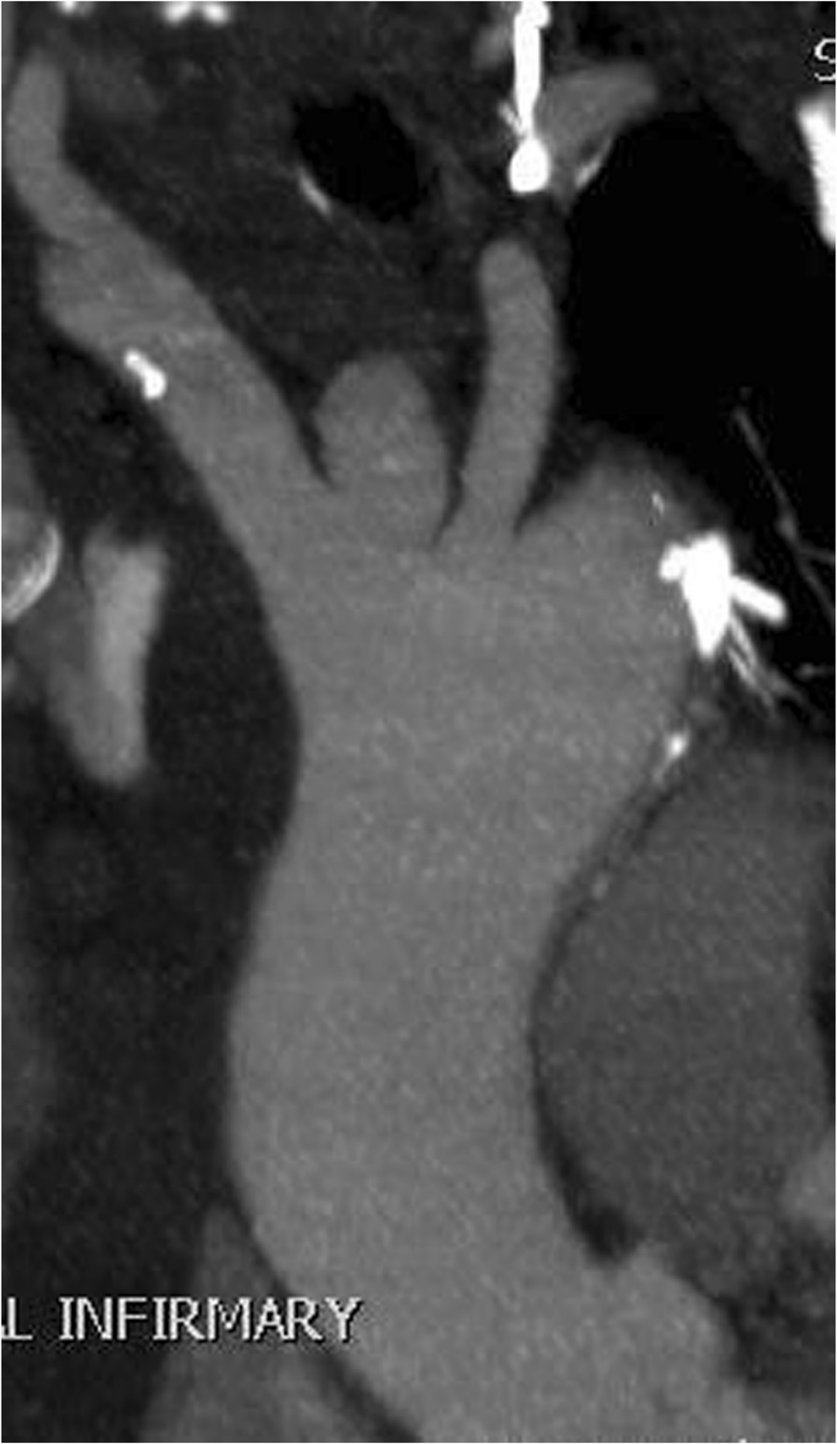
CT scan showing false aneurysm of the innominate artery arising as a blind outpouching at the junction of innominate artery with the arch.
